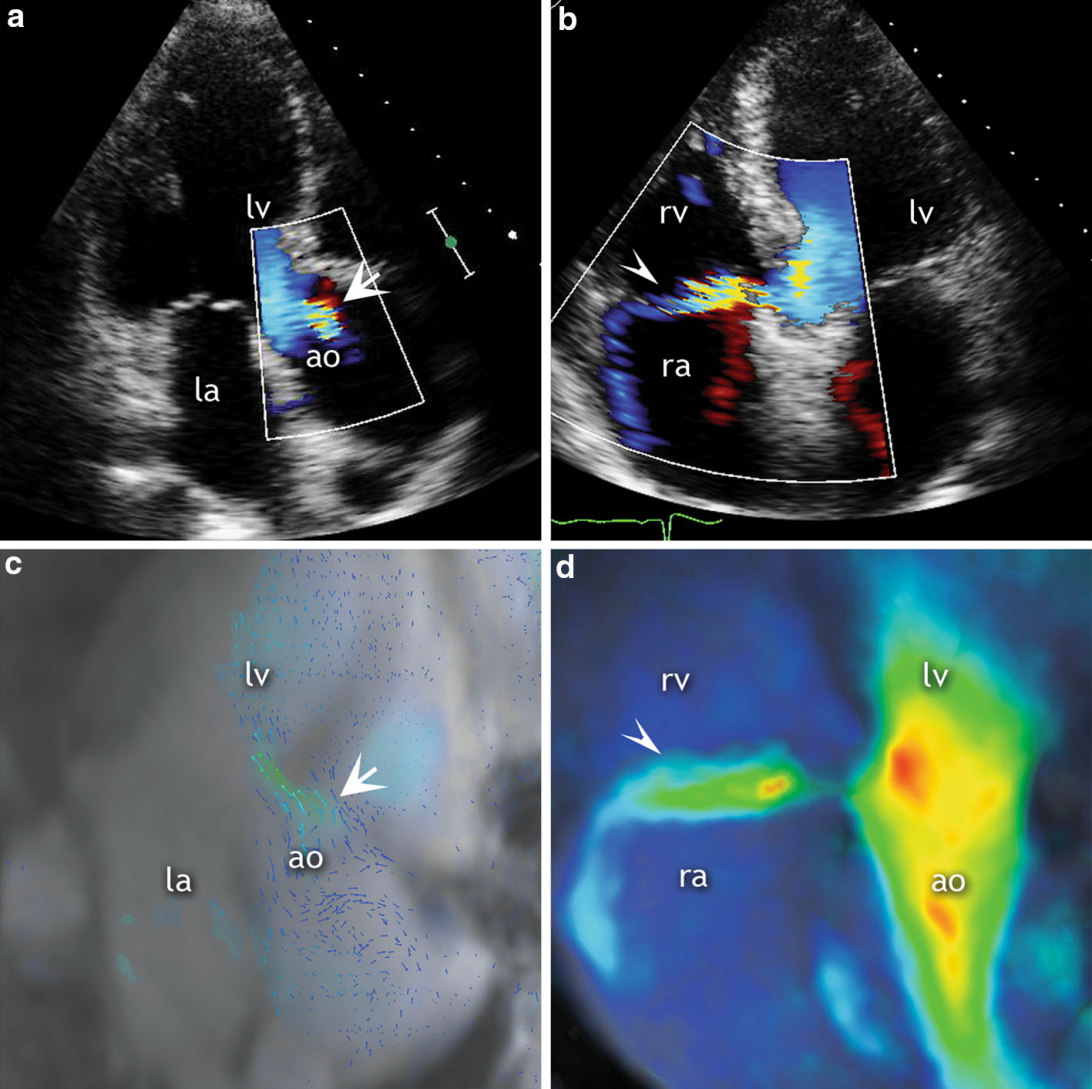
Mild aortic regurgitation and intracardiac shunt. A 47-year-old man post aortic valvotomy and Ross procedure for congenital aortic stenosis. Panel a Three chamber view with color-flow Doppler echocardiography showing a small aortic regurgitant jet (*asterisk*). Panel b Doppler echocardiography imaging, apical four chamber view, zoomed in on the basal septum and the right atrium; color flow through a typical Gerbode defect (*arrows*). Panels c and d CMR 4D flow imaging with corresponding views of the mild aortic regurgitation and the subvalvular, ventriculo-atrial jet, respectively. *lv* left ventricle, *ao* aorta, *ra* right atrium, *rv* right ventricle
